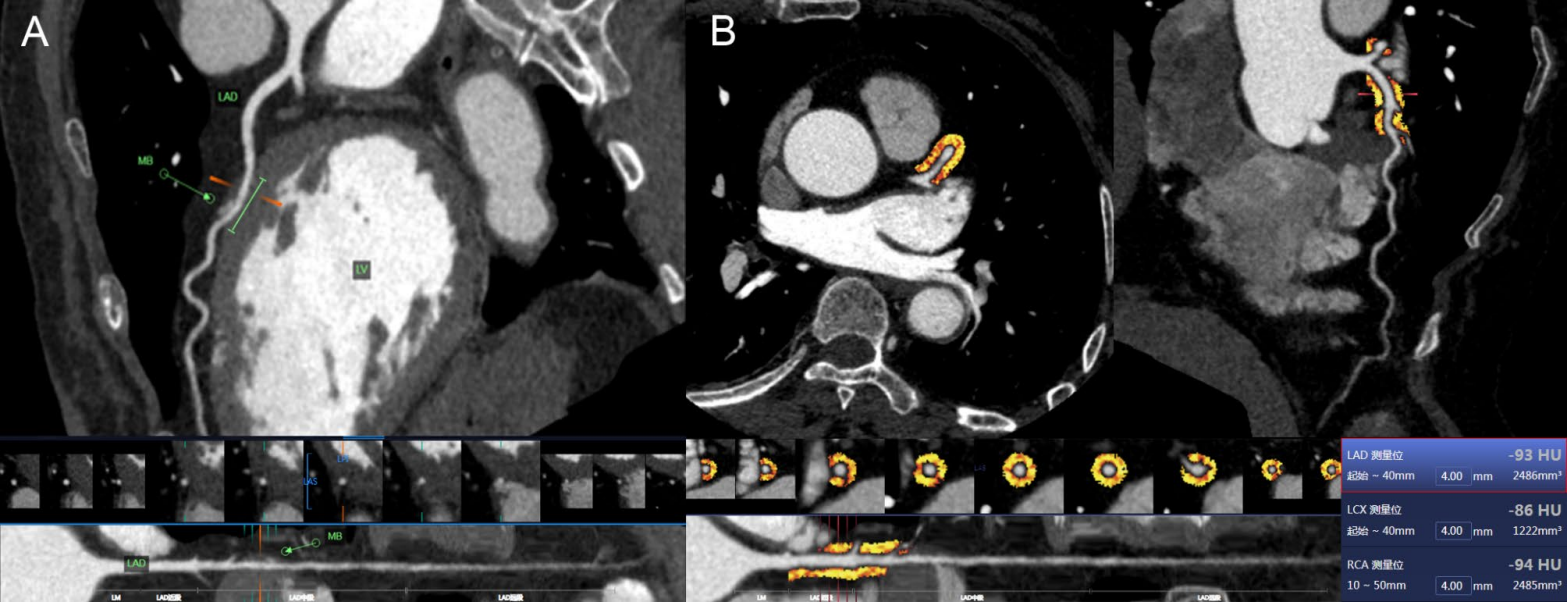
A 62-year-old male underwent CCTA. (a) The patient had an MB on the left anterior descending artery, but coronary artery stenosis covered by the MB was not obvious. The patient had no coronary plaque in the segment proximal to the MB. (b) The FAI_lesion_ value for the 40-mm segment from the coronary artery proximal to the MB was − 93 HU (the red frame). *CCTA*: coronary computed tomography angiography, *MB*: myocardial bridge, *FAI*: fat attenuation index
